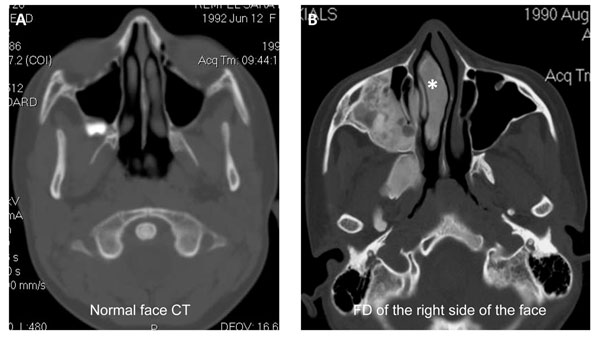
Fibrous dysplasia involving the right maxillary sinus and turbinate. A) Normal facial CT without any FD for comparison. B) FD in the right maxilla and extension into the maxillary sinus. There is also FD involvement of the right turbinate (*) that may explain the patient’s nasal congestion.
